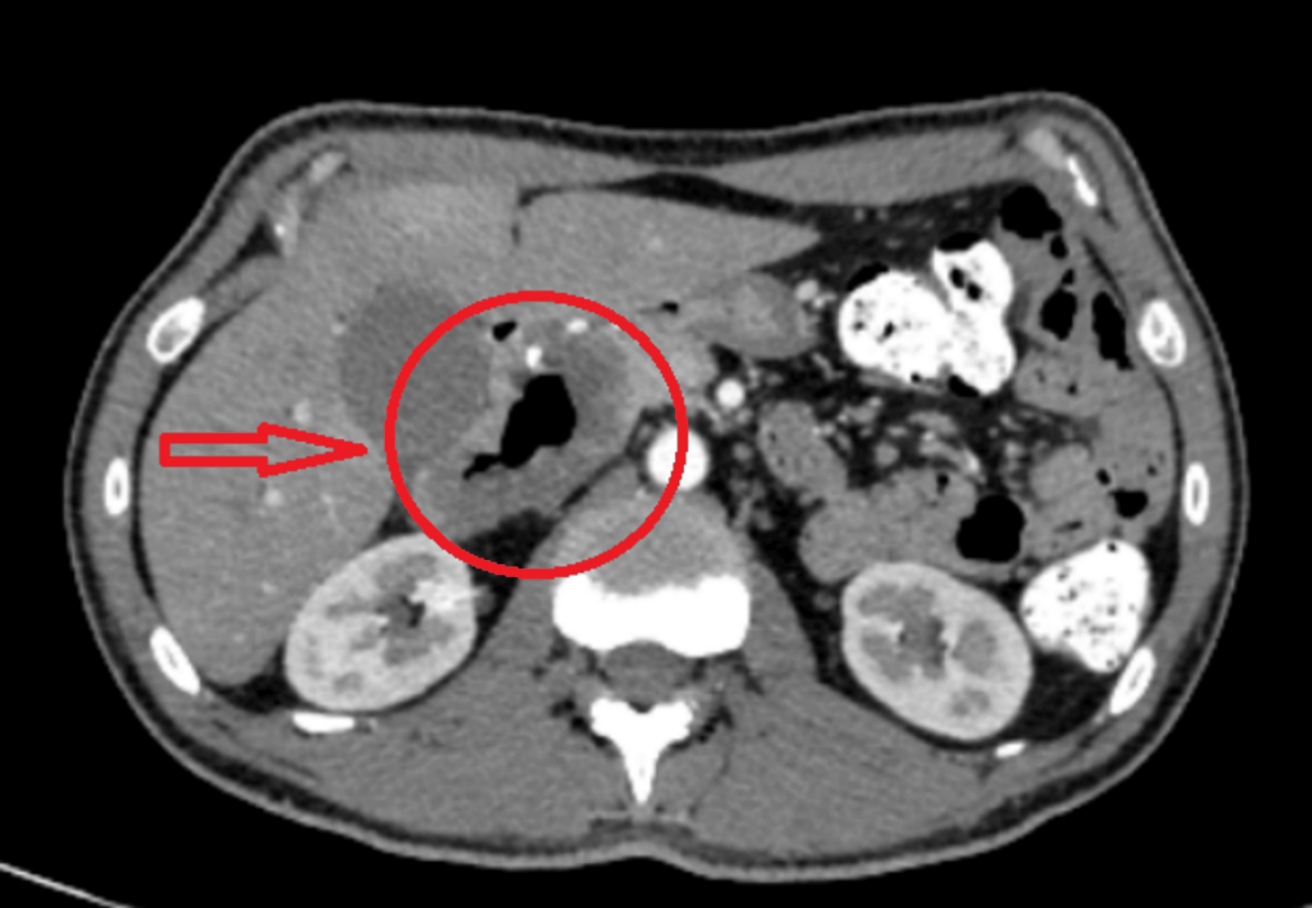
Preoperative CT scan revealing extensive thickening of the duodenal wall
